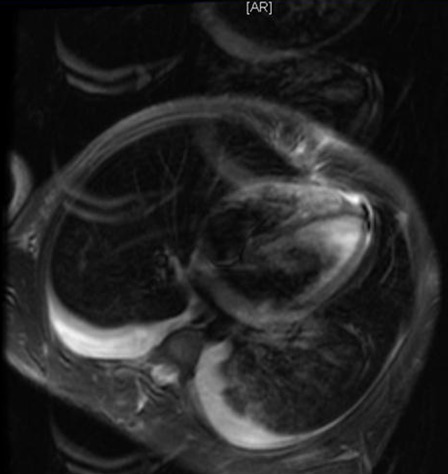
Cardiovascular magnetic resonance imaging performed at day 5 showing hypersignal T2 of the right ventricular
